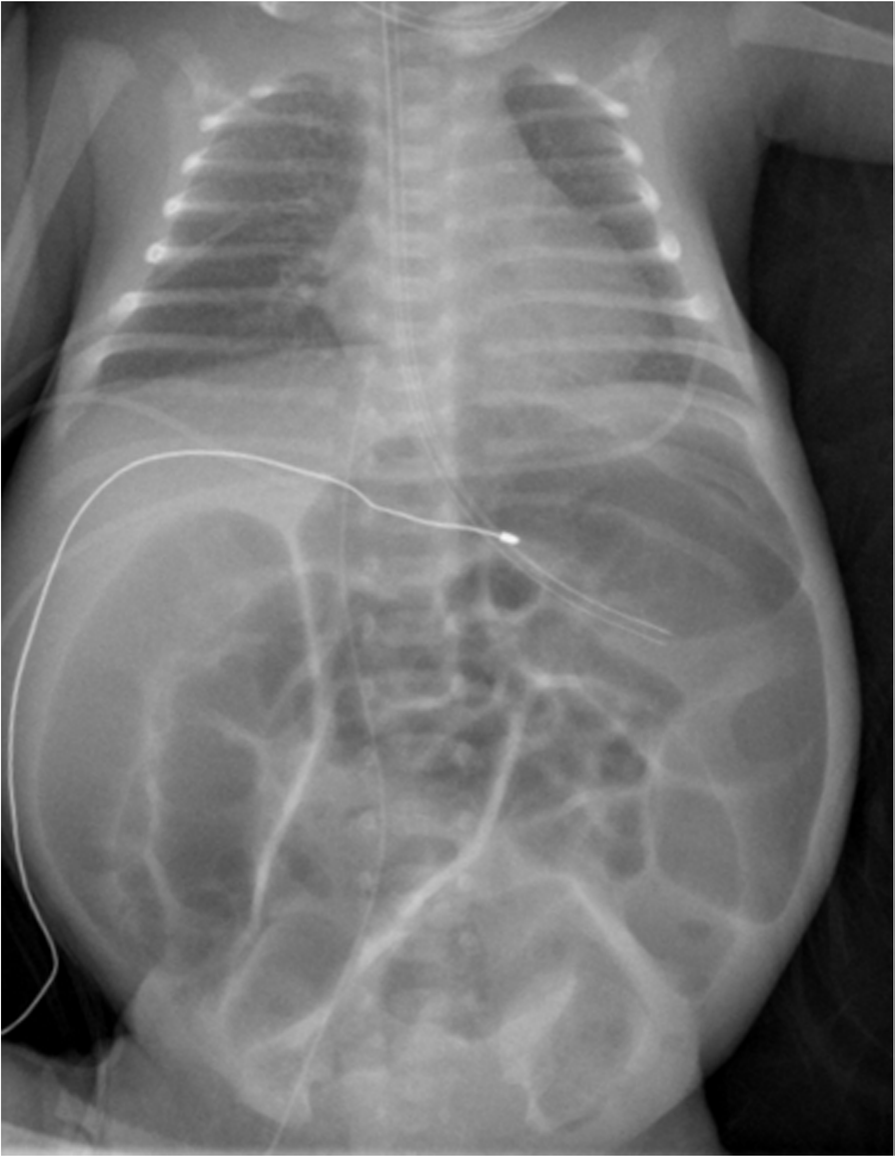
Chest and abdominal X-ray. The X-ray was taken on day of life two before surgery. It shows markedly distended intestinal loops but no sign for perforation or intraluminal gas
